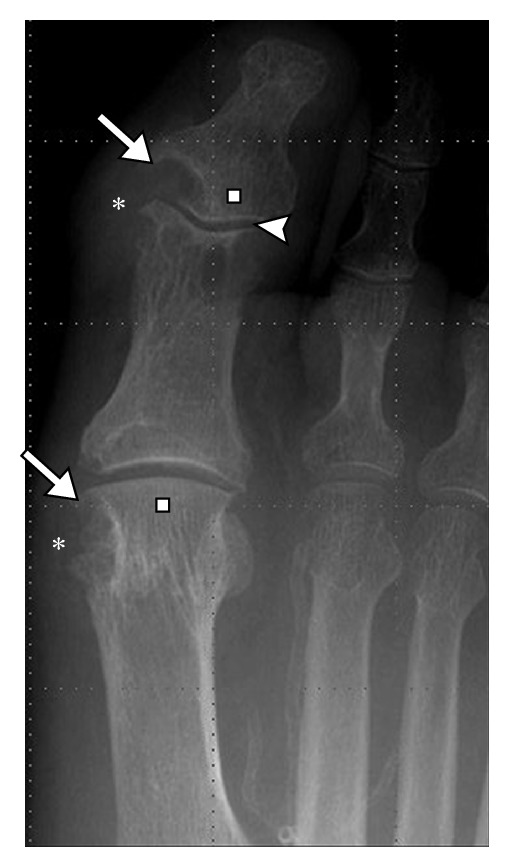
Gout on radiograph. Anteroposterior (AP) view of the 1st metatarsophalangeal (MTP) joint and interphalangeal joint demonstrating juxta-articular erosion with overhanging edge (long arrows). Note the relative preservation of joint space (arrowhead) and subchondral bone density (white square) involving the 1st MTP and interphalangeal joint. *Soft tissue tophus.
